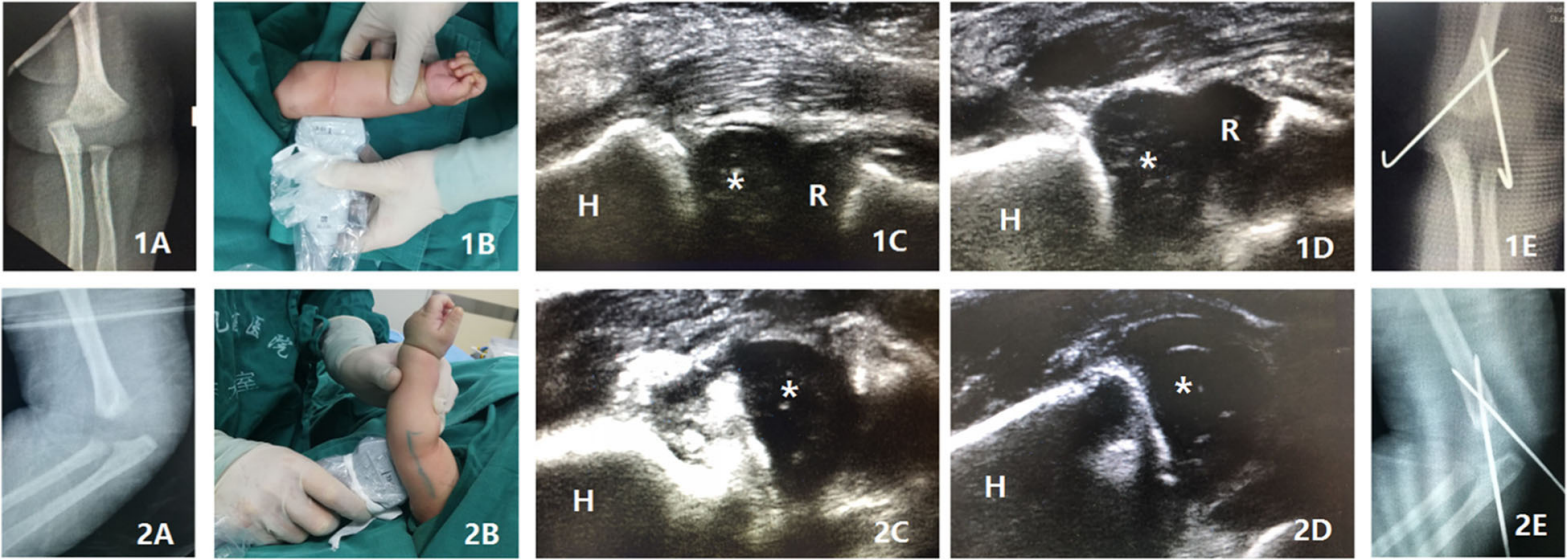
An 9-month-old boy with TFDH secondary to falling down from a bed. The typical medial displacement of the proximal forearm related to the distal humerus on the anteroposterior radiography (1A). The ultrasonic transducer was placed to the radial side of the elbow to assess the lateral displacement of the fracture (1B). The medial displacement of the humeral capitellum (*) and the radial head (R) compared with the humerus (H) (1C). After the closed reduction, the relationship between humeral capitellum (*) and humerus (H) has been corrected (1D). The anteroposterior radiography after treatment (1E). The typical posterior displacement of the proximal forearm related to the distal humerus on the lateral radiography (2A). The transducer was placed to the posterior side of the distal humerus to assess the posterior displacement of the distal humerus (2B). The posterior displacement of the humeral capitellum (*) compared with humerus (H) (2C). After the closed reduction, the posterior displacement of the humeral capitellum (*) has been corrected (2D). The lateral radiography after treatment (2E)
